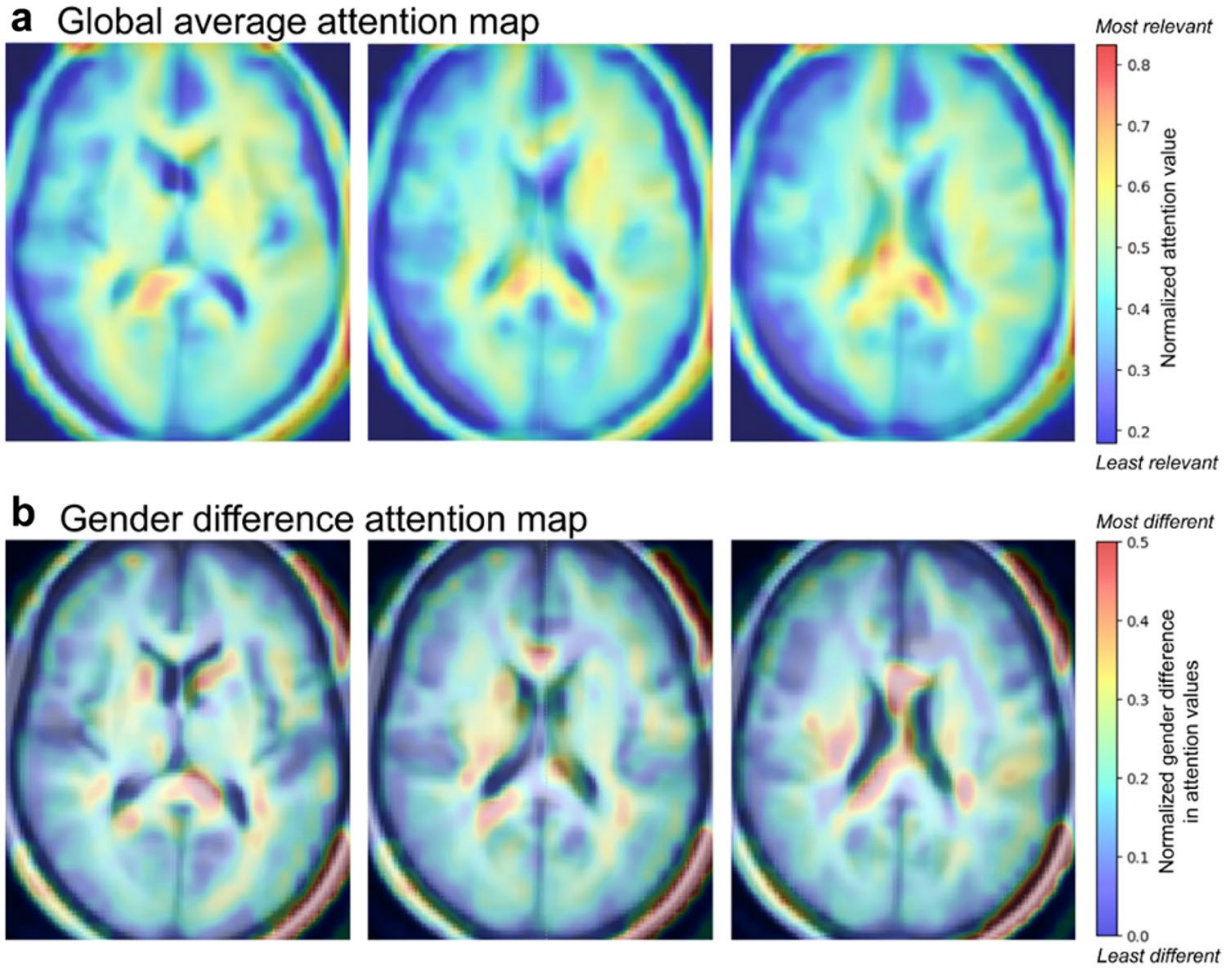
Visualization of critical brain regions for age prediction utilizing Grad CAM. (a) A global average attention map was created from the entire test set (n = 301). Regions marked with higher values, closer to red on the map, signify a greater contribution of those regions to age prediction. (b) A gender difference attention map was generated by subtracting the average attention map of female (n = 153) from that of male (n = 148). Regions marked with higher values, closer to red on the map, indicate stronger gender-specific influences of those regions on age prediction. All attention maps are overlaid over the averaged brain MR image from the test set.
